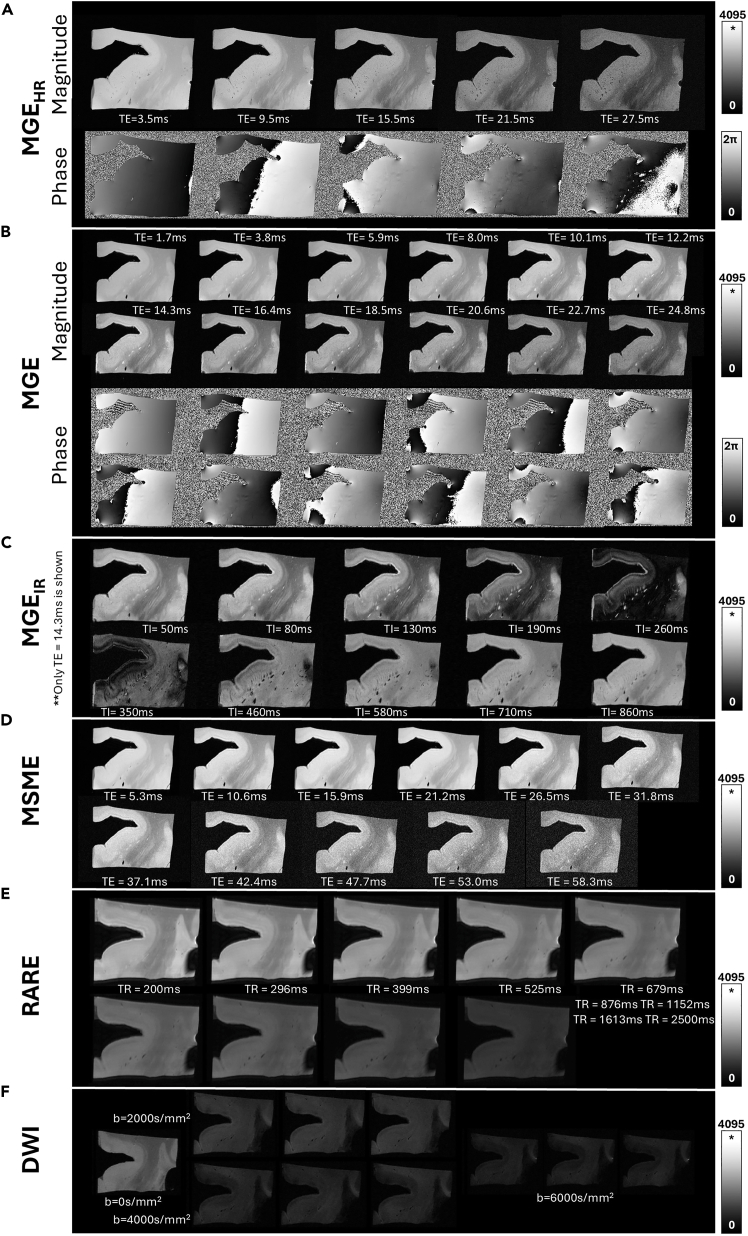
Examples of 9.4T MRI scans of a brain block Exemplary slices of (A) magnitude and phase images of the 5-echo 3D Multi-Gradient-Echo at 70×70×130 μm (MGEHR), (B) magnitude and phase images of the 12-echo 3D Multi-Gradient-Echo at 130×400×130 μm (MGE), (C) magnitude images of Inversion-Recovery 3D MGE (MGEIR) at 130×400×130 μm shown for TE = 14.3 ms at all different inversion times, (D) magnitude images of the 11-echo Multi-Slice Multi-Echo (MSME) at 130×400×130 μm, (E) RApid imaging with Refocused Echoes (RARE) at 130×400×130 μm shown for the different TR values, (F) 3D EPI-based multi-shell Diffusion-Weighted Imaging (DWI) at 200 μm isotropic resolution (one indicative image at b = 0 s/mm^2^ and three for each of the different b-values: 2000, 4000, 6000 s/mm^2^).
